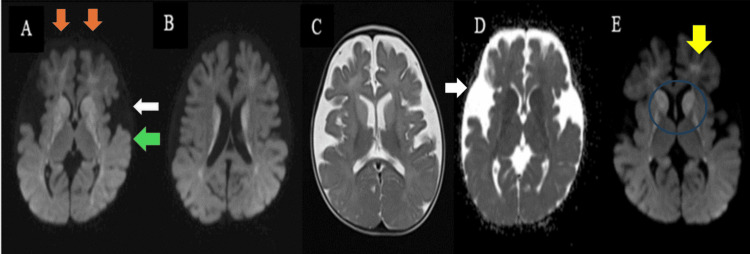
MRI brain axial images (A-D) Bifrontal (indicated by orange arrows) and temporal atrophy are noted (indicated by the green arrow), with prominent sulcal spaces and marked prominence of Sylvian fissures showing bat-wing appearance (indicated by white arrows). (E) A diffuse hyperintense signal is noted in bilateral lentiform nuclei, the head of the caudate nucleus (in blue circle), and bilateral frontal and peritrigonal white matter (indicated by the yellow arrow) on the FLAIR image MRI: magnetic resonance imaging; FLAIR: fluid-attenuated inversion recovery
